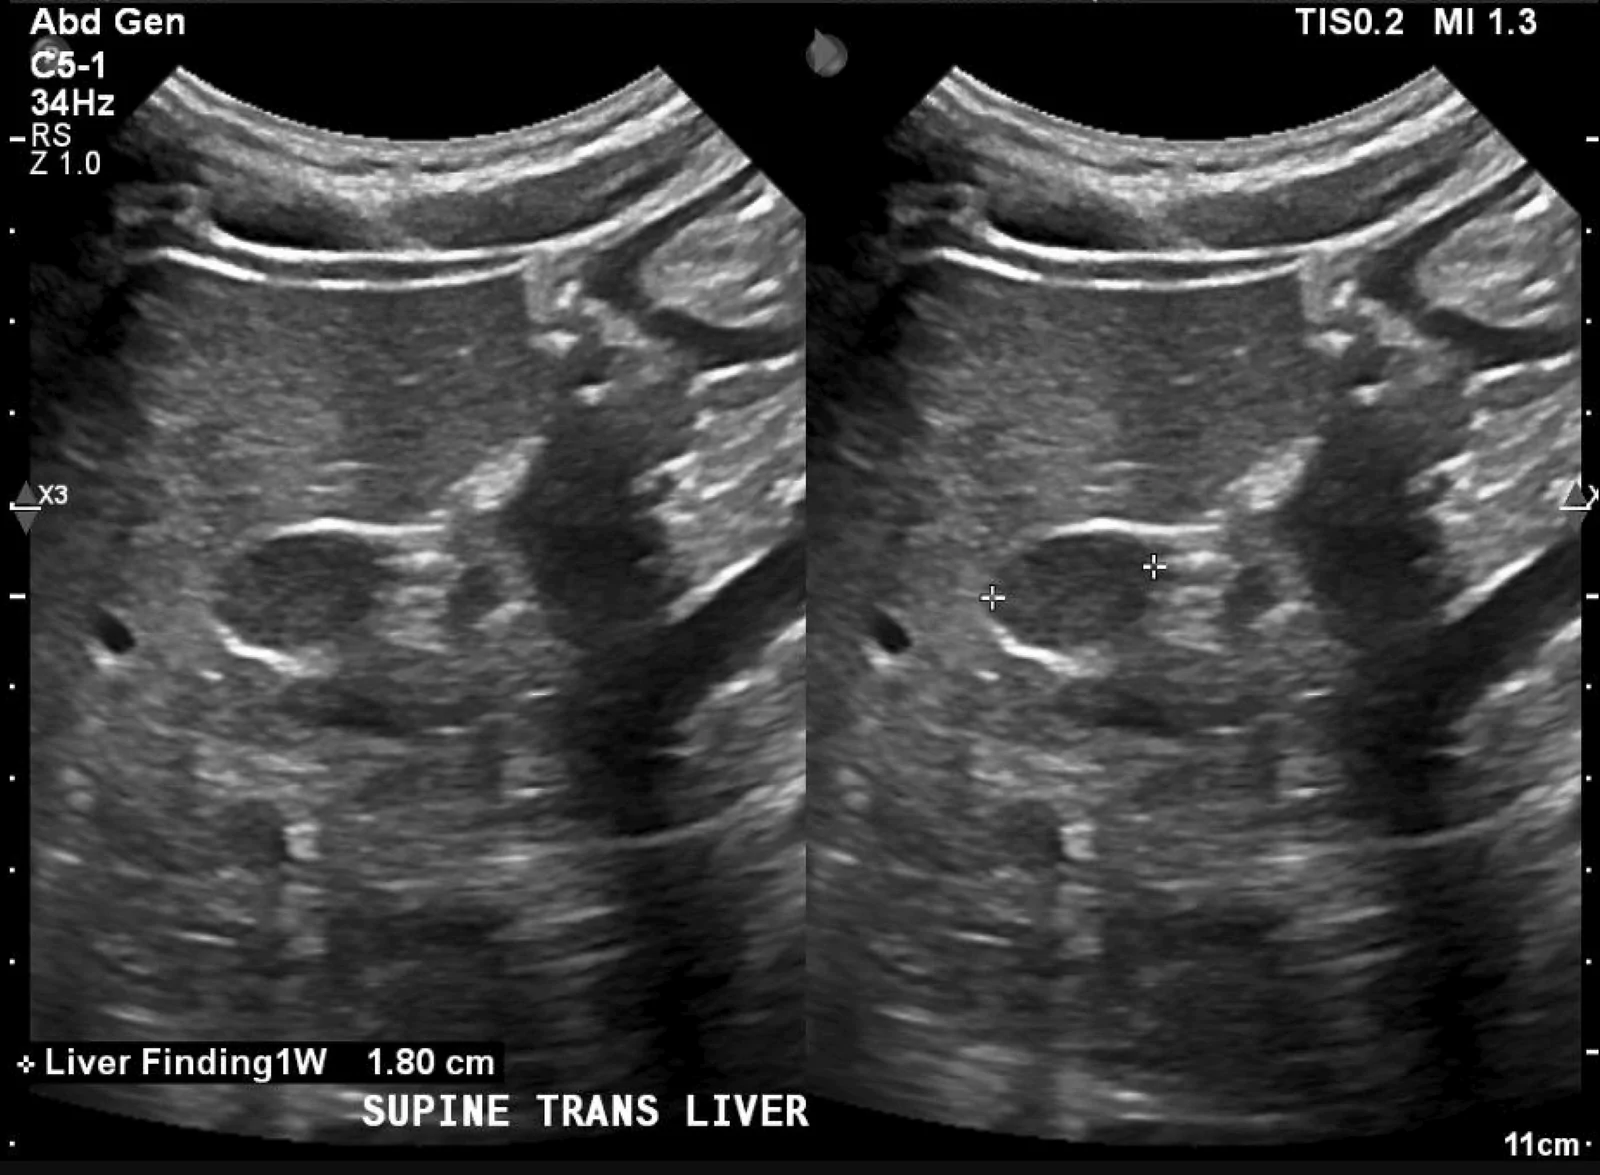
Liver ultrasound revealing a rounded, hypoechoic mass in the hepatic hilum measuring 13 × 13 × 18 mm (width). The liver echotexture is heterogeneous.
